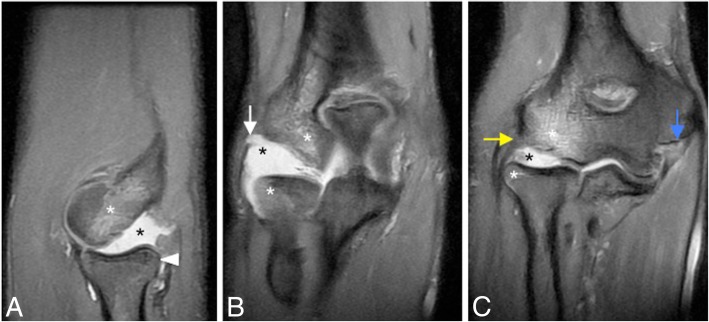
Posterolateral rotatory instability, stage 3B. Sagittal FS PD-weighted MRI (a) and consecutive coronal FS PD-weighted MRI (b, c) showing a posterolateral subluxation of the radial head (white arrowhead), proximal disruption of the lateral ulnar collateral ligament (white arrow), partial proximal disruption of the radial collateral ligament (yellow arrow), proximal disruption of the anterior bundle of the medial collateral ligament (blue arrow), radial head and posterior capitellar contusions (white asterisks), and joint effusion (black asterisks)
